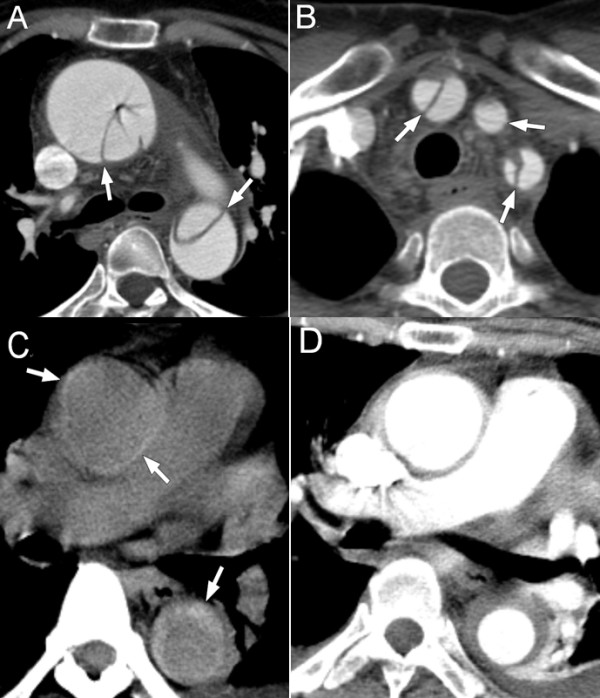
Dissection type. (A) Classic type A aortic dissection with visible intimal flap (arrow) inside dilated ascending and descending aorta. (B) Dissected supra-aortic branches (innominate, left common carotid and left subclavian artery) in the same patient as in figure A. (C) Type A intramural hematoma with crescent or circular high attenuation (arrow) in ascending and descending aortic wall on noncontrast CT. (D) No contrast enhancement of the thickened arotic wall in the same patient as in figure C.
